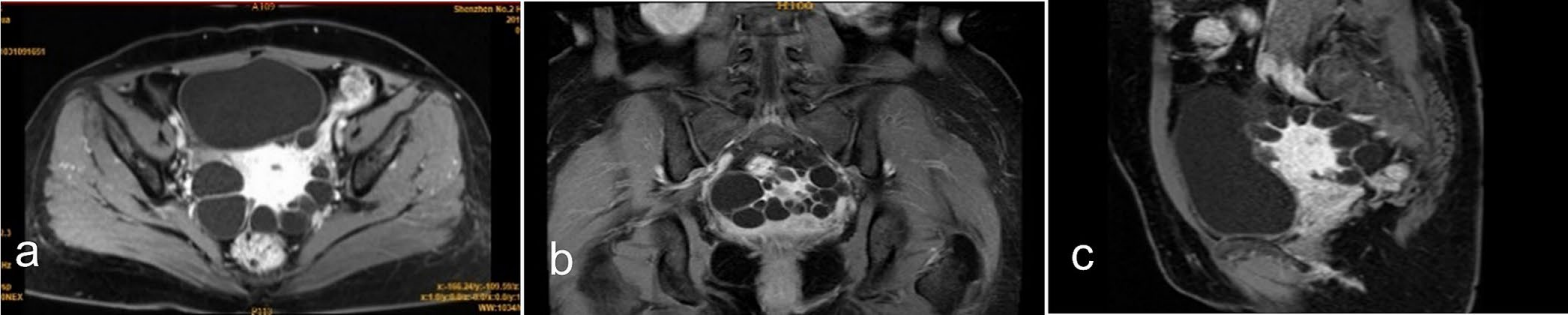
Contrast-enhanced MRI findings: (a) Axial view, (b) coronal view, and (c) sagittal view show the lesion located in the uterine myometrium near the serosal layer. The cyst wall exhibits mild enhancement compared to the uterine myometrium, while no enhancement is observed within the cystic content.
